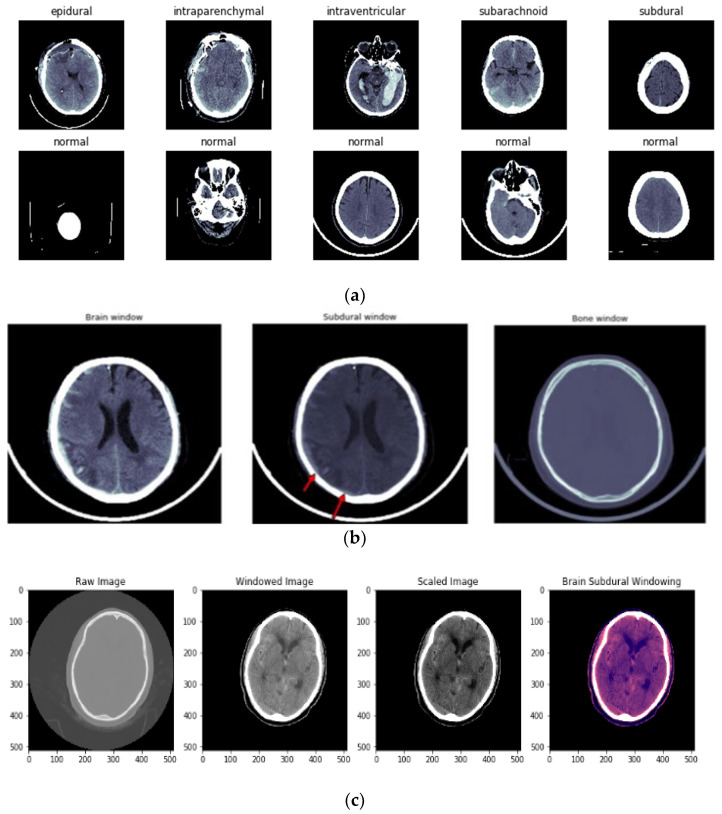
(a) Different types of ICH and normal images before windowing is applied. (b) Types of windowing used in preprocessing of ICH. First image shows the brain window, second image represents the subdural window, and third image indicates the bone window. (c) Image preprocessing using the windowing technique. First image shows the raw CT image, second image shows the windowing technique applied over the raw image, third image is a scaled image, and final image is brain subdural window applied image.
